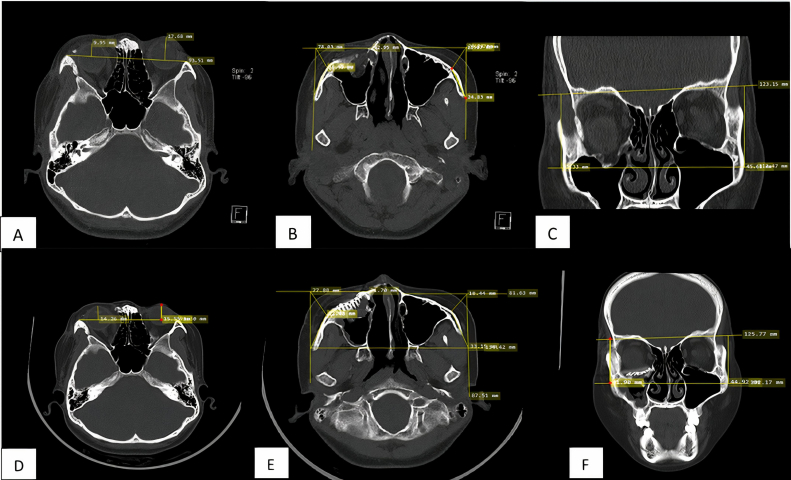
(A) Enophthalmos examination on preoperative CT scan shows enophthalmos in the left eye (with a difference of >2 mm between the two eyes). (B) Malar projection deficit examination. There is a deficit between the fracture side and the normal of 2.86 mm. (C) Malar height deficit examination. The difference is 4.35 mm compared to the normal side. (D) Enophthalmos examination on postoperative CT-scan shows improvement of enophthalmos in the right eye (with a difference of< 2mm between the two eyes). (E) Malar projection deficit examination shows decreasing deficit between the fracture side and the normal of 1,13mm. (F) Malar height deficit examination shows decreasing deficit between the fracture side and the normal of 3.02mm.
